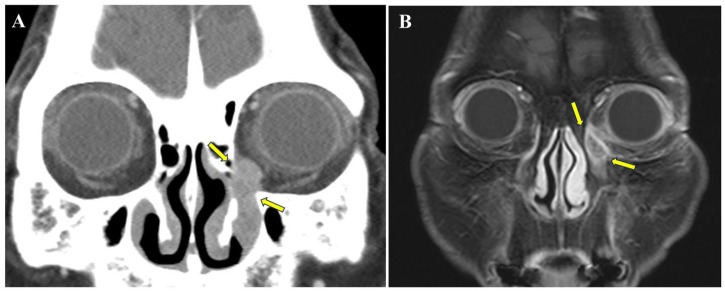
Preoperative imaging findings of the nasolacrimal duct melanoma. (A) Contrast-enhanced coronal orbital computed tomography (CT) scan in soft tissue window reveals an elongated, homogenously enhancing tumor lesion (yellow arrows) occupying the left medial canthal region and extending into the nasolacrimal duct. Note the widening of the lacrimal bony canal without gross bony destruction. (B) Coronal T1-weighted fat-suppressed contrast-enhanced magnetic resonance imaging (MRI) of the head and neck demonstrates a heterogeneously enhancing tumor lesion (yellow arrows) involving the left medial canthus and the upper portion of the nasolacrimal duct, corresponding to the CT findings.
